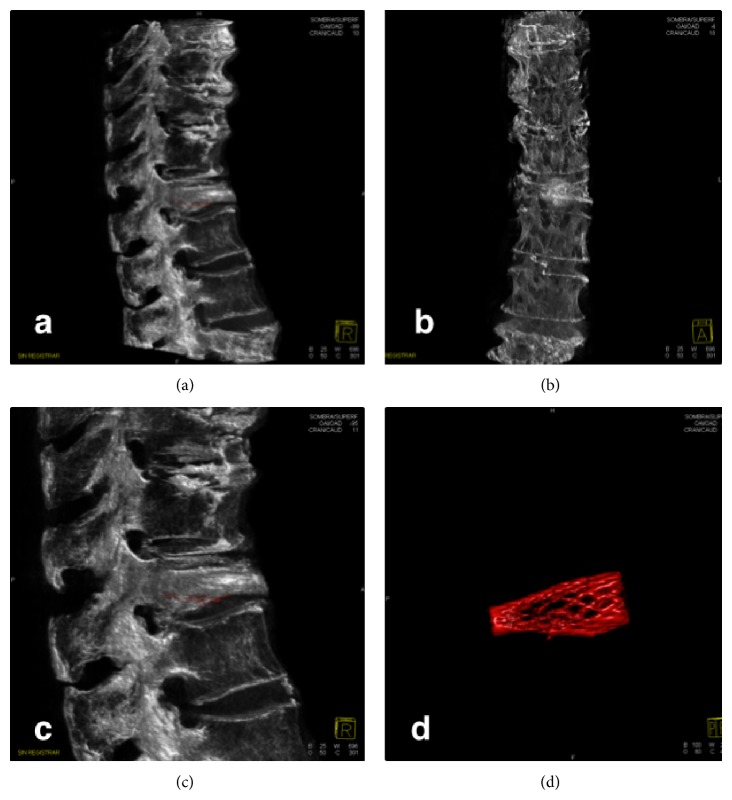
Flat panel detector computed tomography allowed visualization of the disposal of cement inside the affected vertebrae in all angles ((a), (b), and (c)) and the detailed characterization of the intravertebral device (d).
